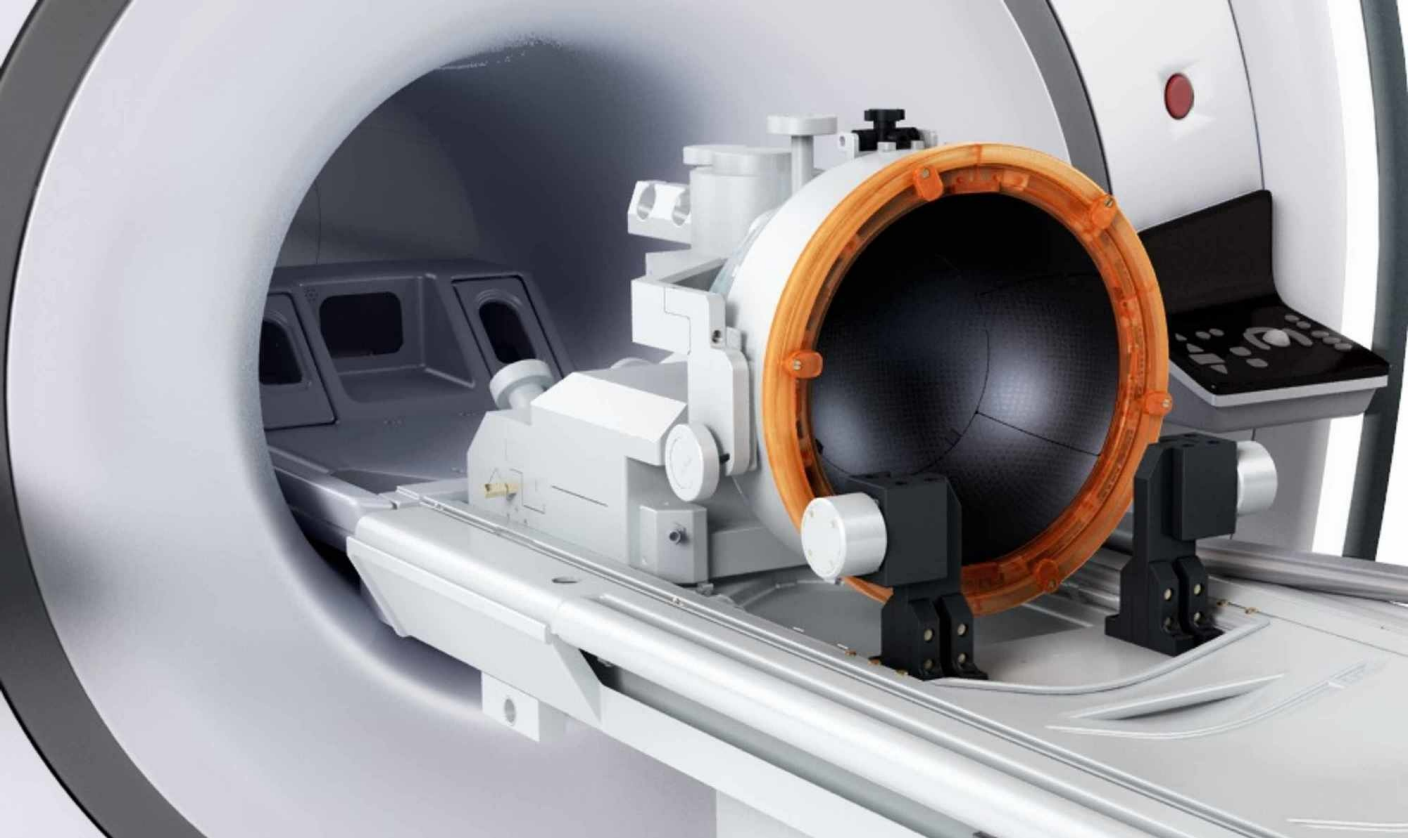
The Phase array, piezoelectric helmet shaped high-intensity focused ultrasound transducer with 1024 elements at a frequency of 650 KHz. (Credit: Image obtained from INSIGHTEC Ltd.)
